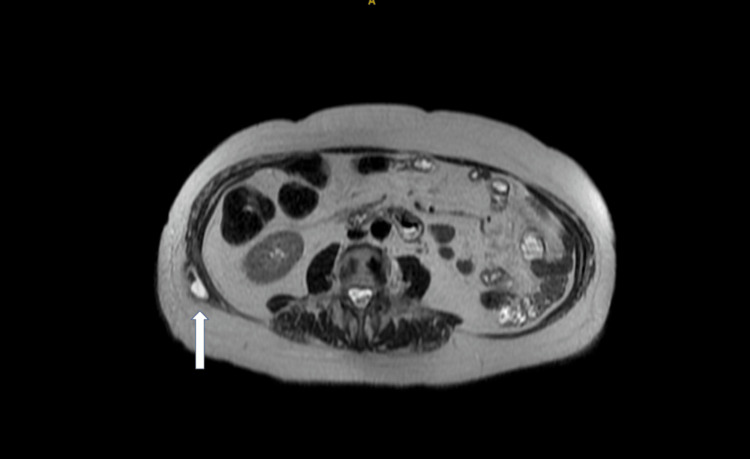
MRI showing 3 x 1 cm fluid collection in the right oblique muscle MRI, magnetic resonance imaging
